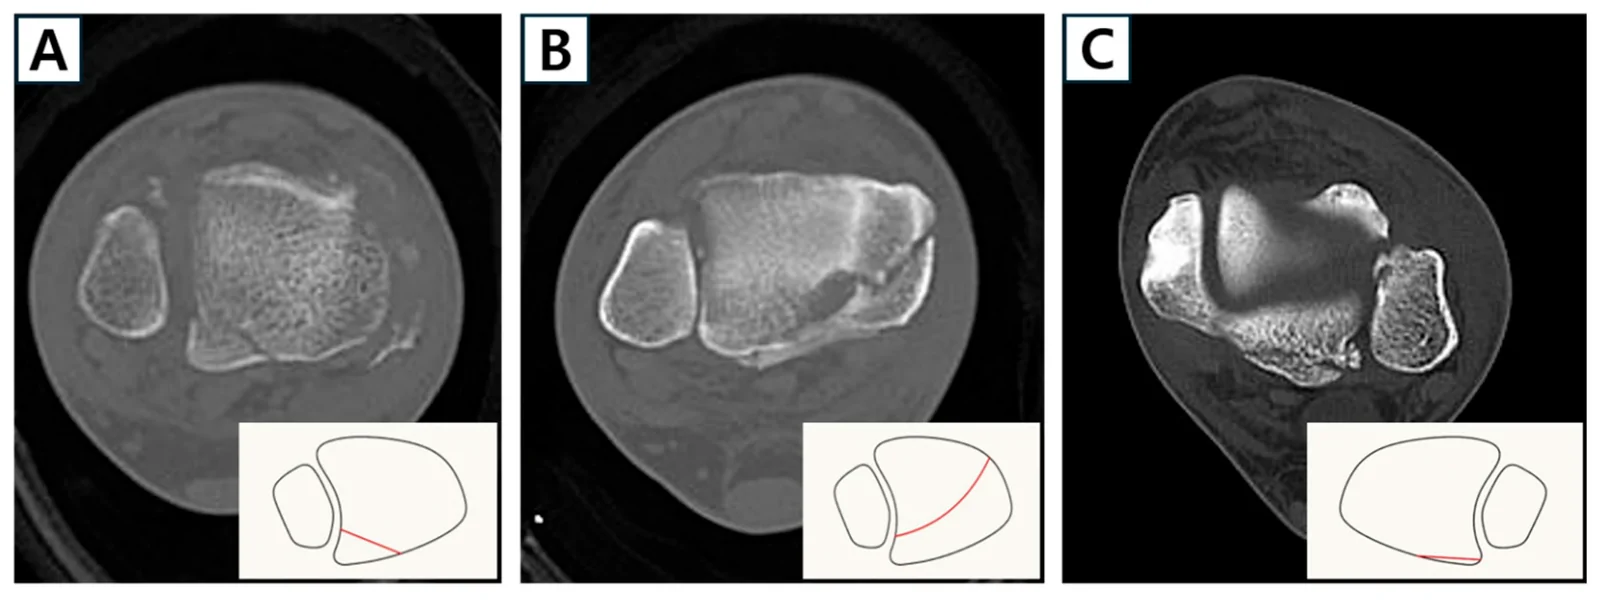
CT-based classification of posterior malleolar fractures according to the Haraguchi classification. (A) Haraguchi type I, wedge-shaped posterolateral fragment; (B) Haraguchi type II, medial-extension fracture; and (C) Haraguchi type III, small shell fracture.
